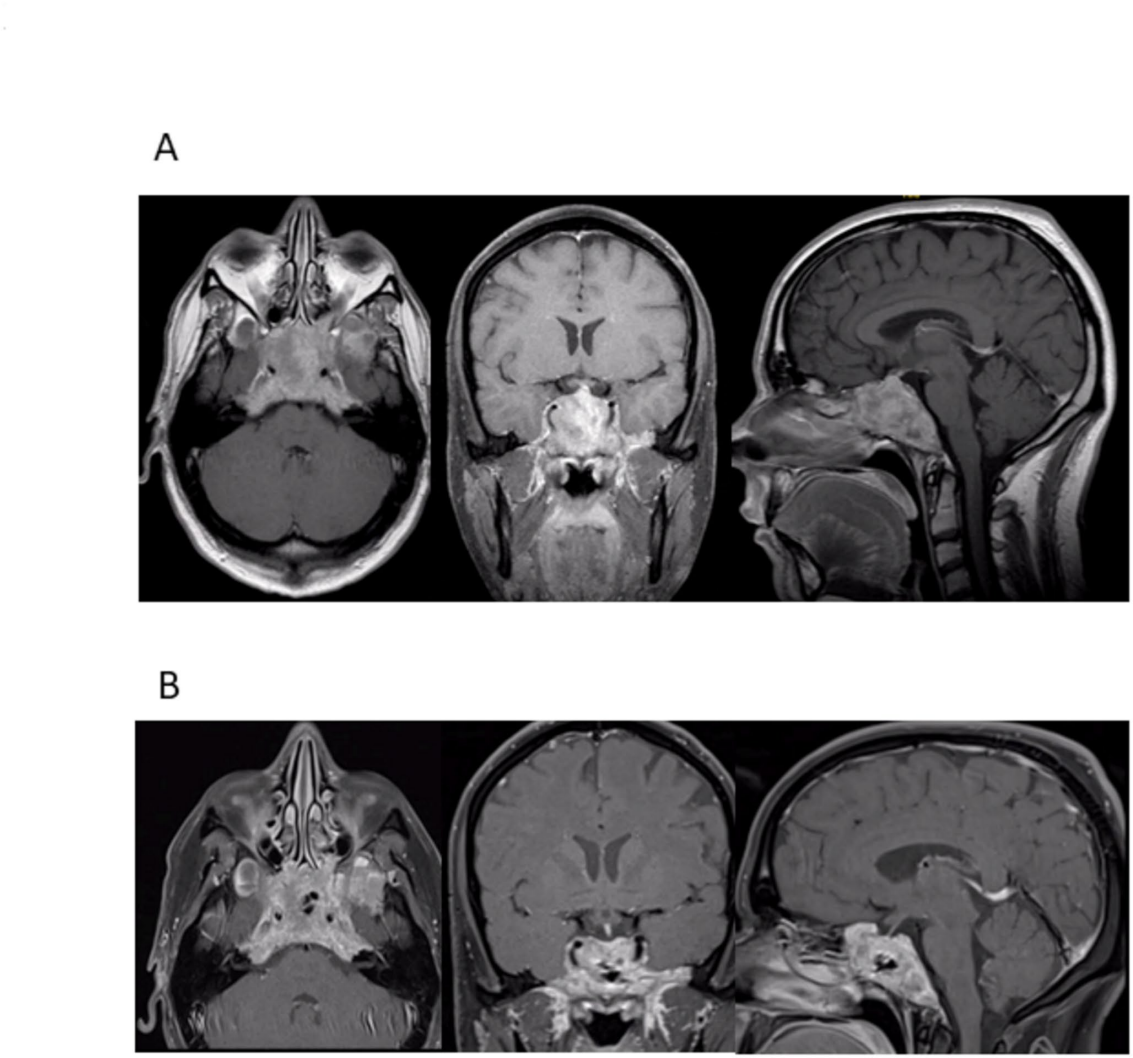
Imaging studies of patient 2 (34-year-old man). A T1-weighted contrast enhanced MRI studies show a giant prolactinoma with a maximum diameter of 8 cm encasing both ICAs and invading the cavernous sinus and the clivus. B T1-weighted contrast enhanced MRI studies 10 days after initiation of cabergoline therapy. There is massive tumor shrinkage with a small depot of air in the center of the tumor
